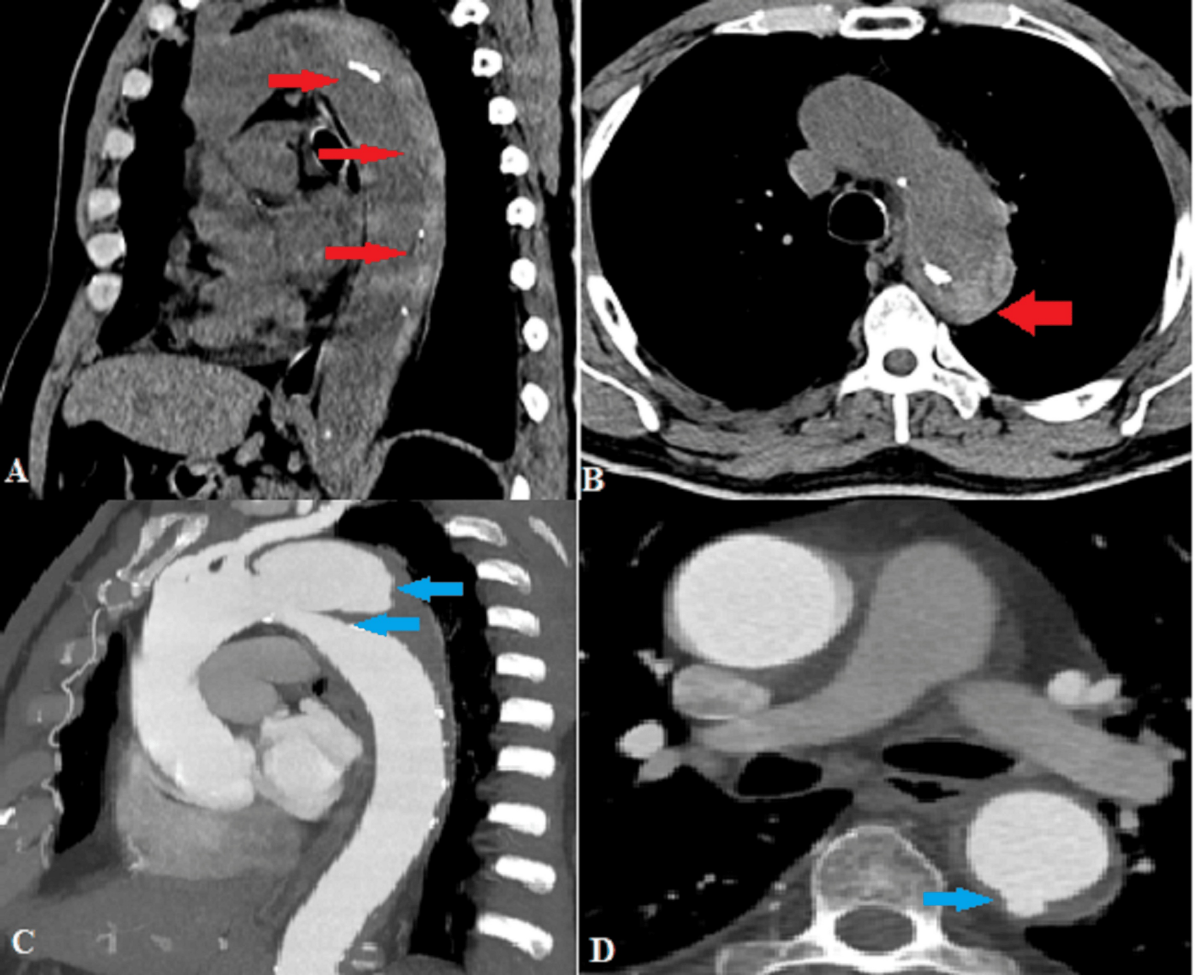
(A) Sagittal and (B) axial non-contrast CT images demonstrate crescentic hyperdensity along the posterior walls of the arch of the aorta and descending aorta (red arrows). Intimal calcifications surround the true lumen. (C) Sagittal CT angiography image shows an intramural hematoma complicated by an ulcer-like projection (blue arrows). (D) Axial CT angiography shows a contrast-filled ulcer crater in the posterior wall of the descending aorta (blue arrow).
